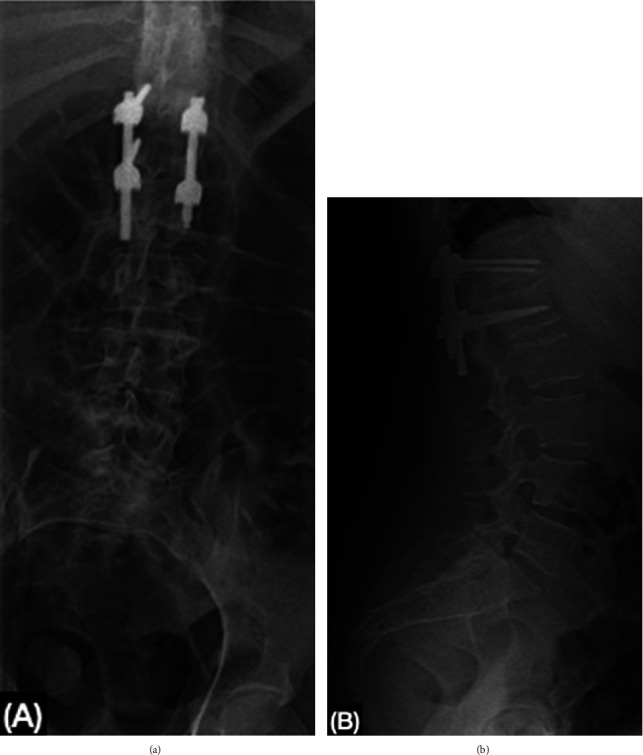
Postoperative x-ray of the lumbar spine: (a) transpedicular screws and rod are seen between T12 and L1. The form and height of vertebral bodies are preserved; (b) appropriate amplitude of inter somatic space. A lumbar angle of 57° indicates physiological lordosis.
